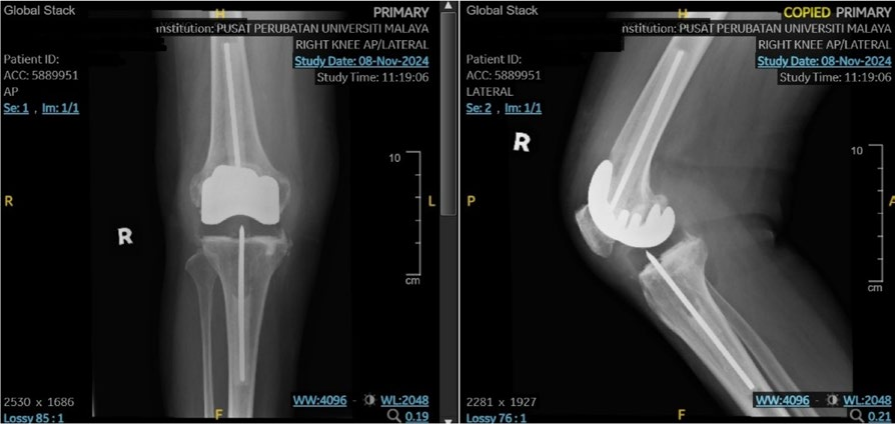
Postoperative radiographs of a patient post-explantation and placement of an articulating spacer for PJI
